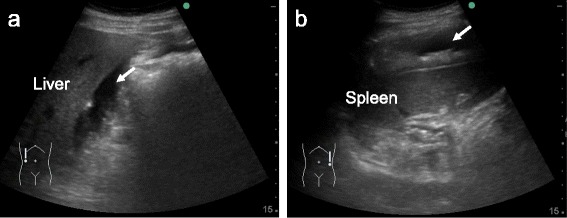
Ultrasound images in a 47-year-old man who presented with left upper continuous abdominal pain. The patient began to feel pain after heavy physical labor without awareness of a traumatic event. Bedside ultrasound after history taking and a physical examination revealed free fluid in Morison’s pouch (a, *arrow*), perisplenic space (b, *arrow*), and rectovesical pouch. Contrast-enhanced computed tomography showed hemoperitoneum and splenomegaly with a low-density, striped area in the lower pole. He was diagnosed as having splenic rupture and treated conservatively
